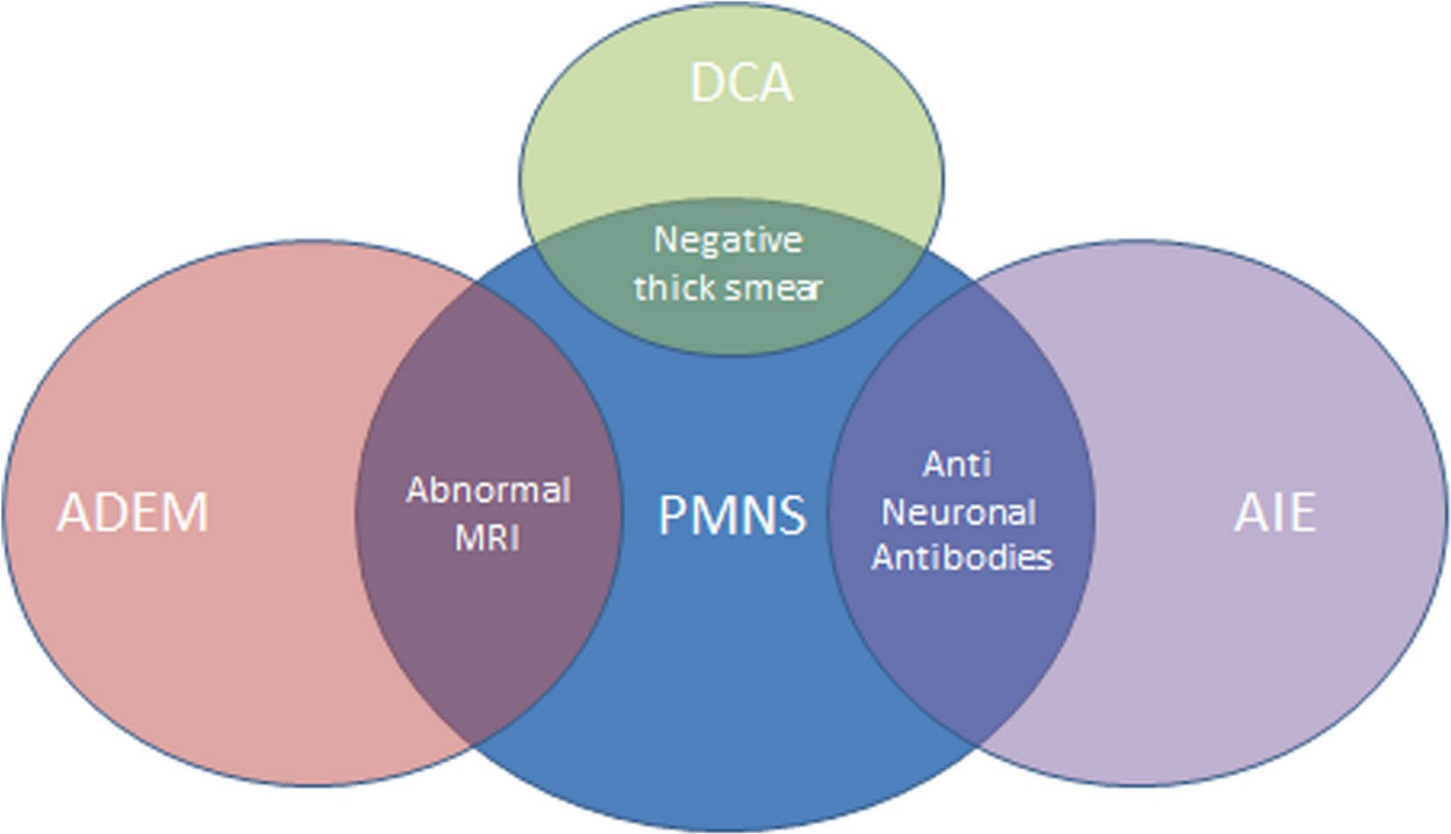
Nosological framework for post-malaria neurological syndrome. DCA is difficult to classify in PMNS because in most of the published cases, data on parasite clearance was lacking. However, other elements (delay, lack of sensitivity to antimalarials) advocate for at least a relationship between them. Delayed post-infectious cerebellar involvement was described in a number of the confirmed PMNS cases; MRIs were normal for some and ADEM-compatible for others. ADEM: acute diffuse encephalomyelitis. *MRI* magnetic resonance imaging, *DCA* diffuse cerebellar ataxia, *AIE* autoimmune encephalitis, *PMNS* post-malaria neurological syndrome
